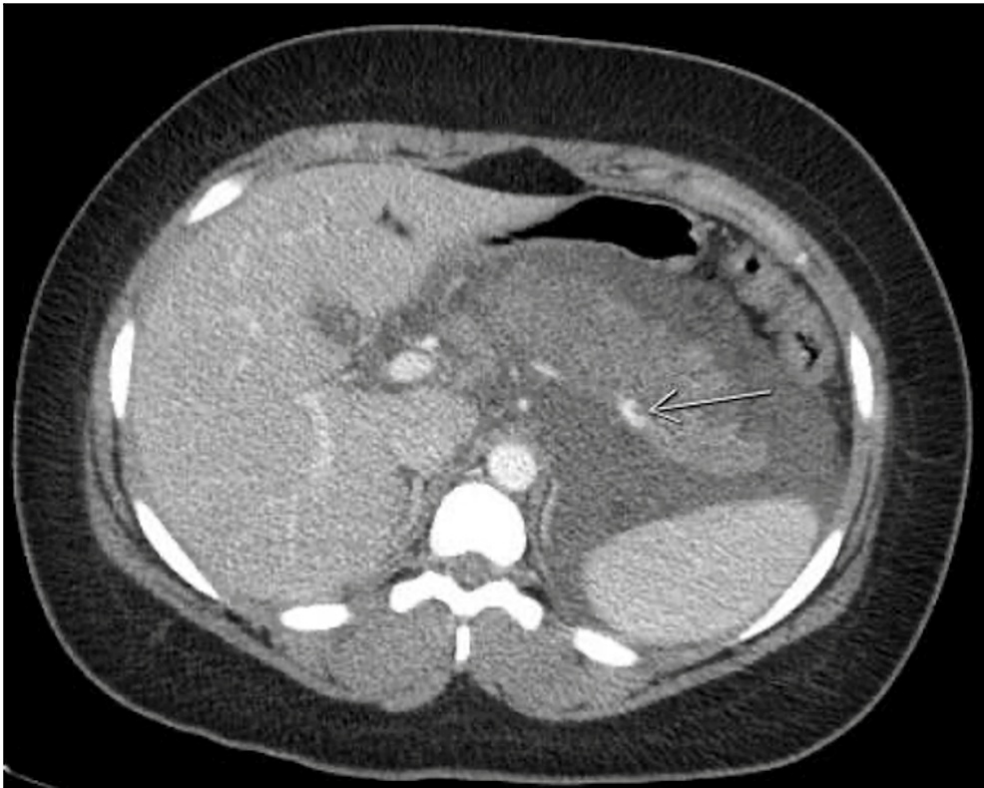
A repeat CT of the abdomen and pelvis (axial view) with contrast, performed two days after the first CT showed worsening pancreatitis with signs of liquefactive necrosis associated with left greater than right basilar subsegmental consolidation and showed interval narrowing of the splenic vein with nonocclusive thrombus.
